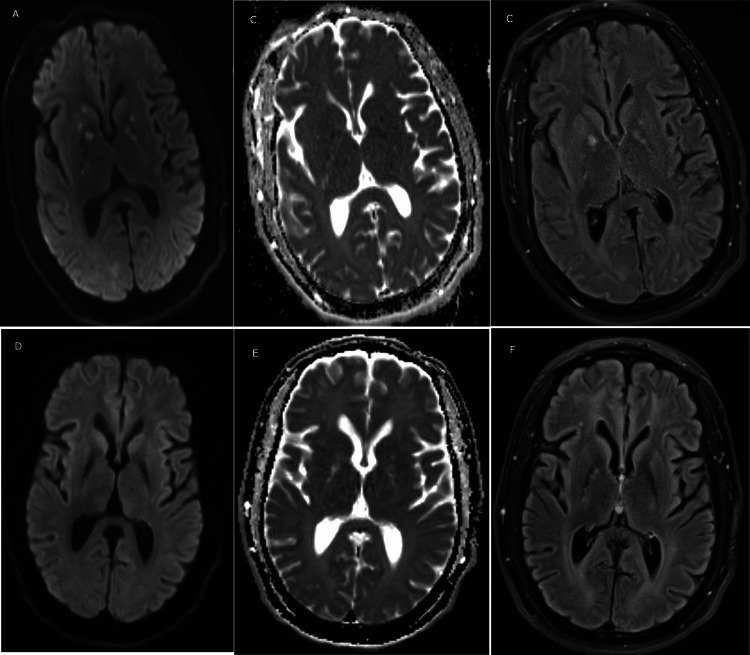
MRI images of the bilateral basal ganglia at presentation and at the three-month follow-up ADC/DWI images show diffusion restriction and T2 FLAIR shows edema of the bilateral basal ganglia at presentation and at the three-month follow-up A. Diffusion-weighted imaging (DWI) image at presentation; B. Apparent diffusion coefficient (ADC) image at presentation; C. T2 fluid-attenuated inversion recovery (FLAIR) image at presentation; D. DWI image at three months; E. ADC image at three months; F. T2 FLAIR image at three months.
